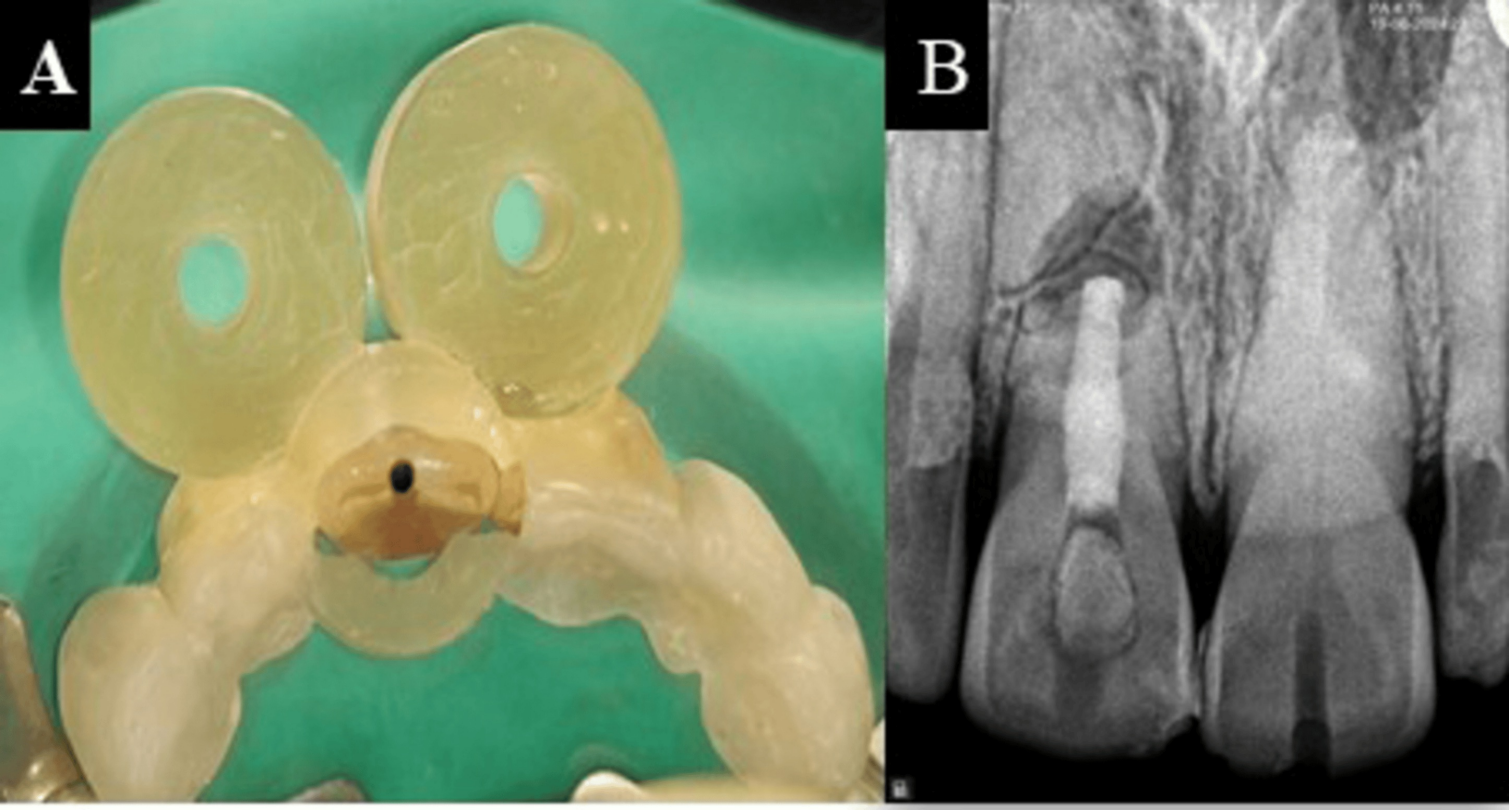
(A) Minimally invasive access opening on the tooth and (B) minimally invasive access opening radiograph
